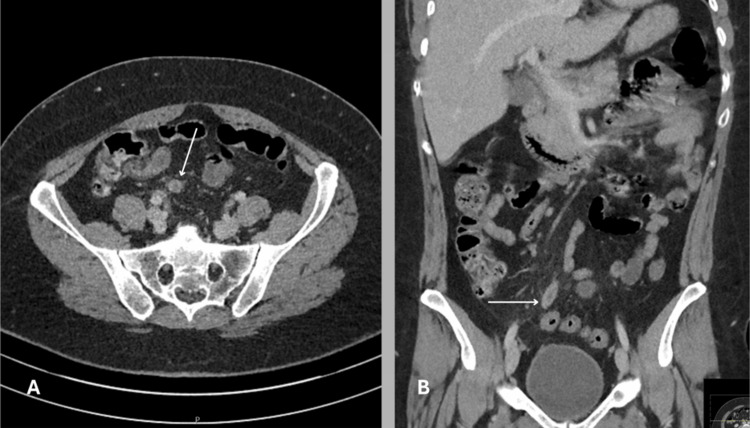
CT scan of a 25-year-old female from the study showing classic signs of acute appendicitis. Typical CT findings in appendicitis include a distended, thick-walled appendix with surrounding fat stranding indicating peri-appendiceal inflammation. A) Axial cross-sectional view showing the inflamed appendix with fat stranding (arrow). B) Coronal view confirming the same finding (arrow). Image Credit: The figure is original and derived from the data collected in the present study.
